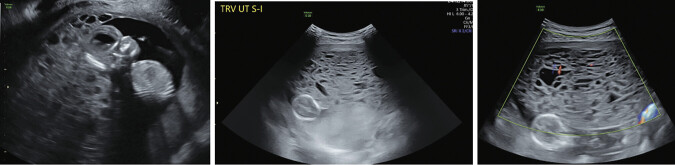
Transvaginal ultrasound of partial hydatidiform mole with classic “snowstorm” appearance.
